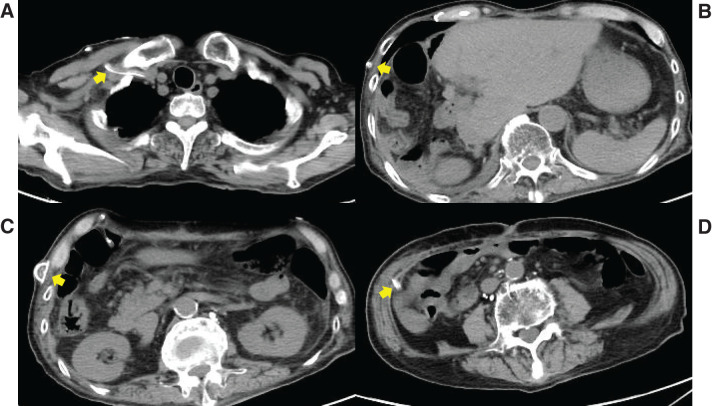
CT findings after placement of a peritoneovenous shunt (Denver shunt). Ascites was diminished after shunting. The shunt tube entered the right subclavian vein (A) from the lower abdominal cavity (D) via the subcutis (B, C).
